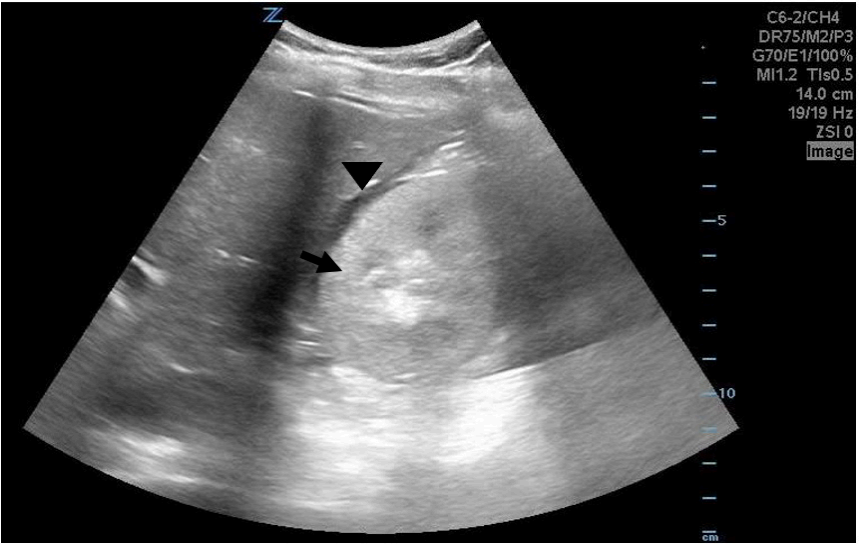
Point-of-care ultrasound of the right kidney seen in the transverse plane
